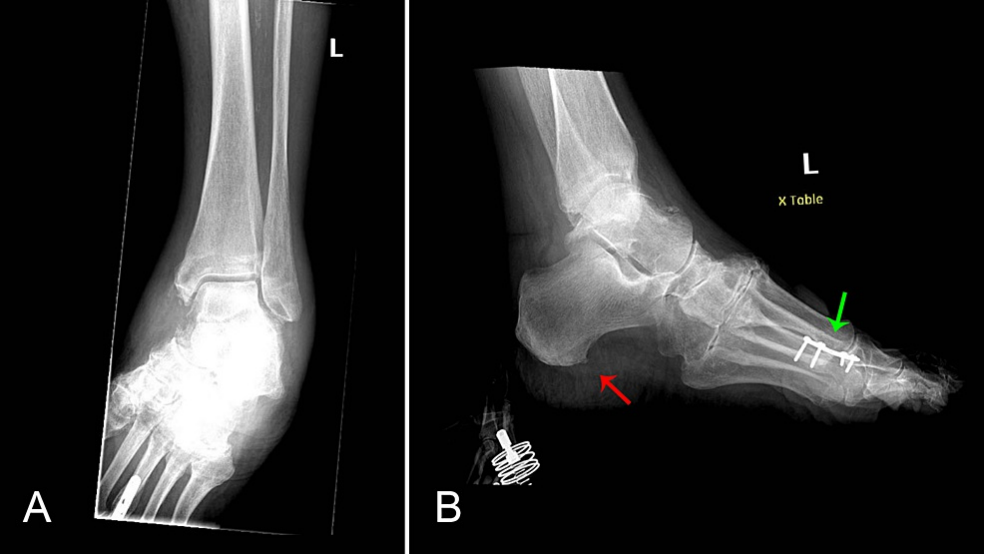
X-rays of the left foot (A) and left ankle (B) showing a metallic plate with screws along the distal diaphysis of the third metatarsal bone (green arrow), a small calcaneal spur (red arrow), some osteopenia, degenerative changes in tarsal metatarsal joints, and soft-tissue swelling.
